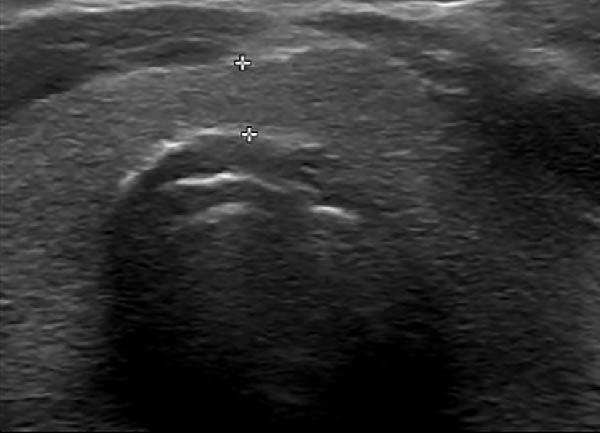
Ultrasound of the neck in transverse view showing a left sided hypoechoic nodule.
